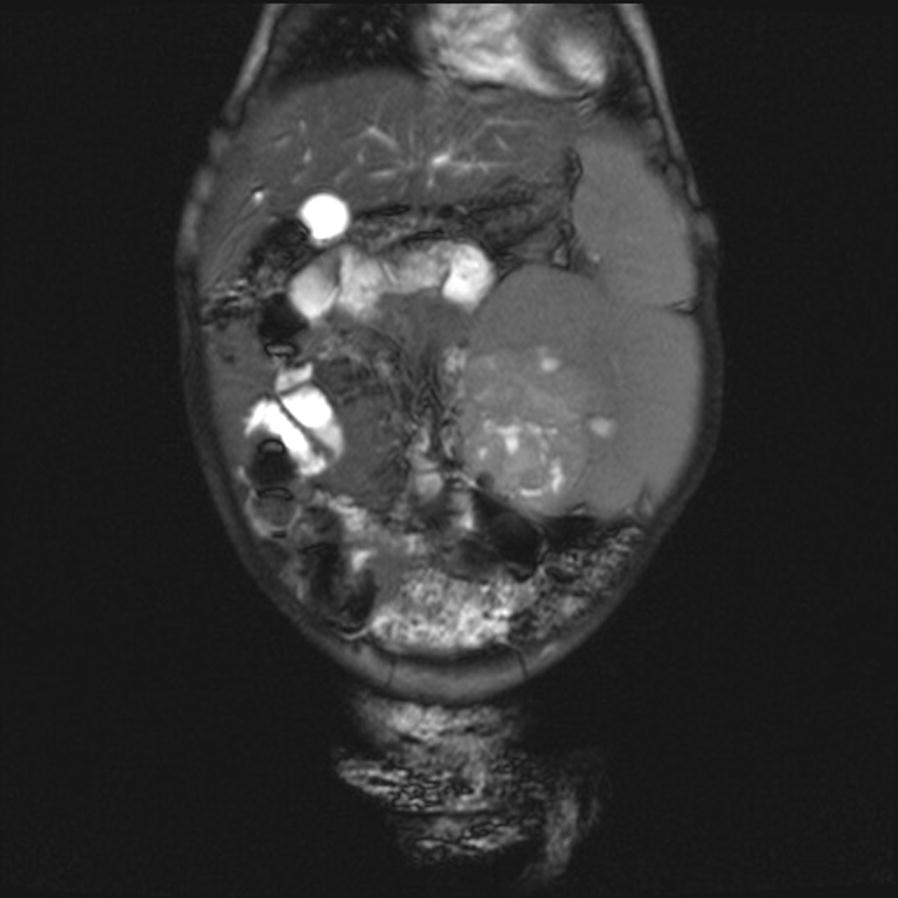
Coronal slice of T1 gadolinium enhanced MRI performed after 6 weeks of chemotherapy revealing a 6 × 6.3 cm heterogeneous enhancing lesion in the medial aspect of the left kidney with multiple linear and round tiny cysts. A dominant gadolinium enhancing 1 cm area to the left of this heterogeneous mass is suspicious for complicating Wilms tumor
